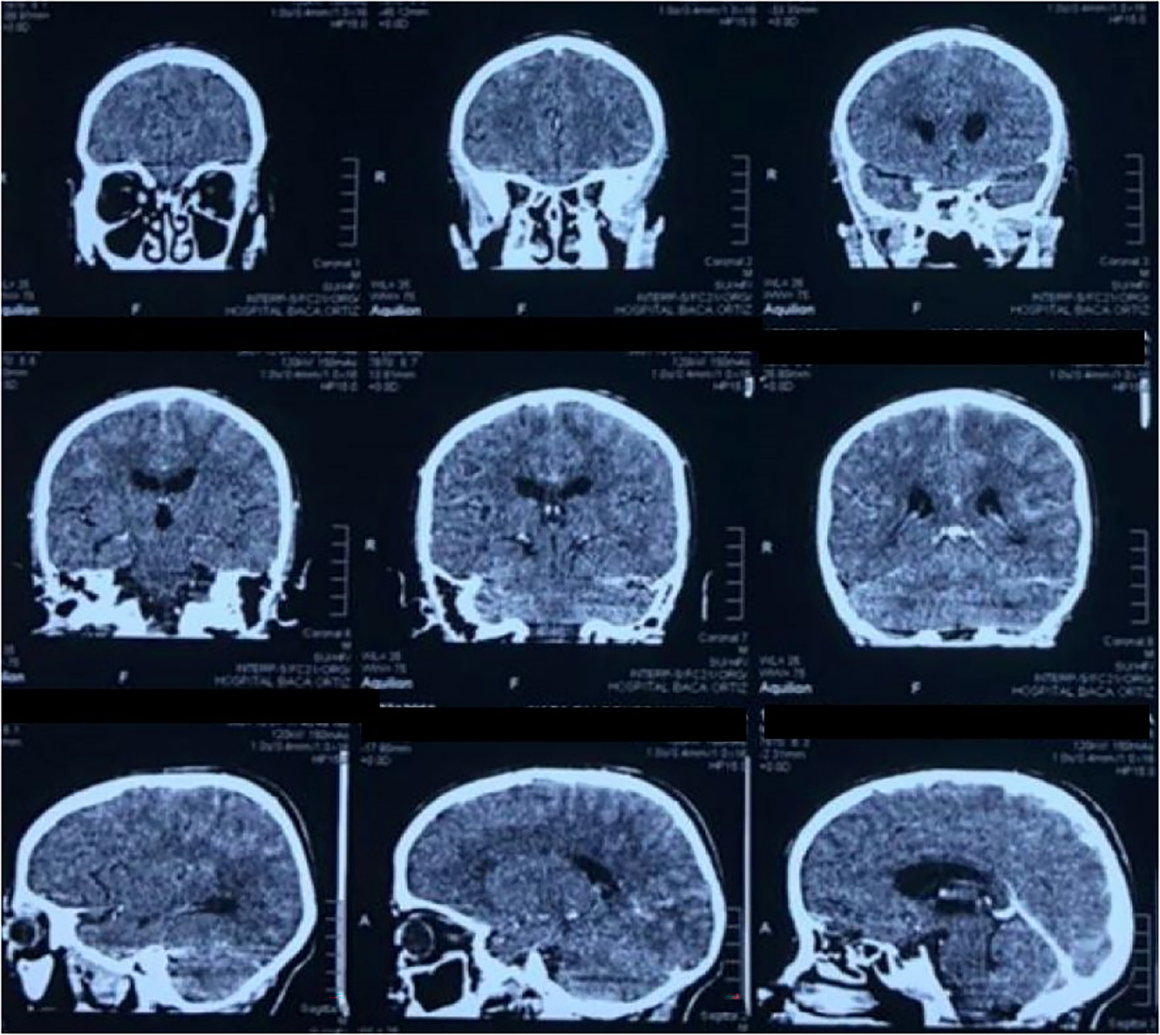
CT scan of the brain in coronal and sagittal planes shows a ventricular system with preserved morphology. No meningeal enhancement is observed.
